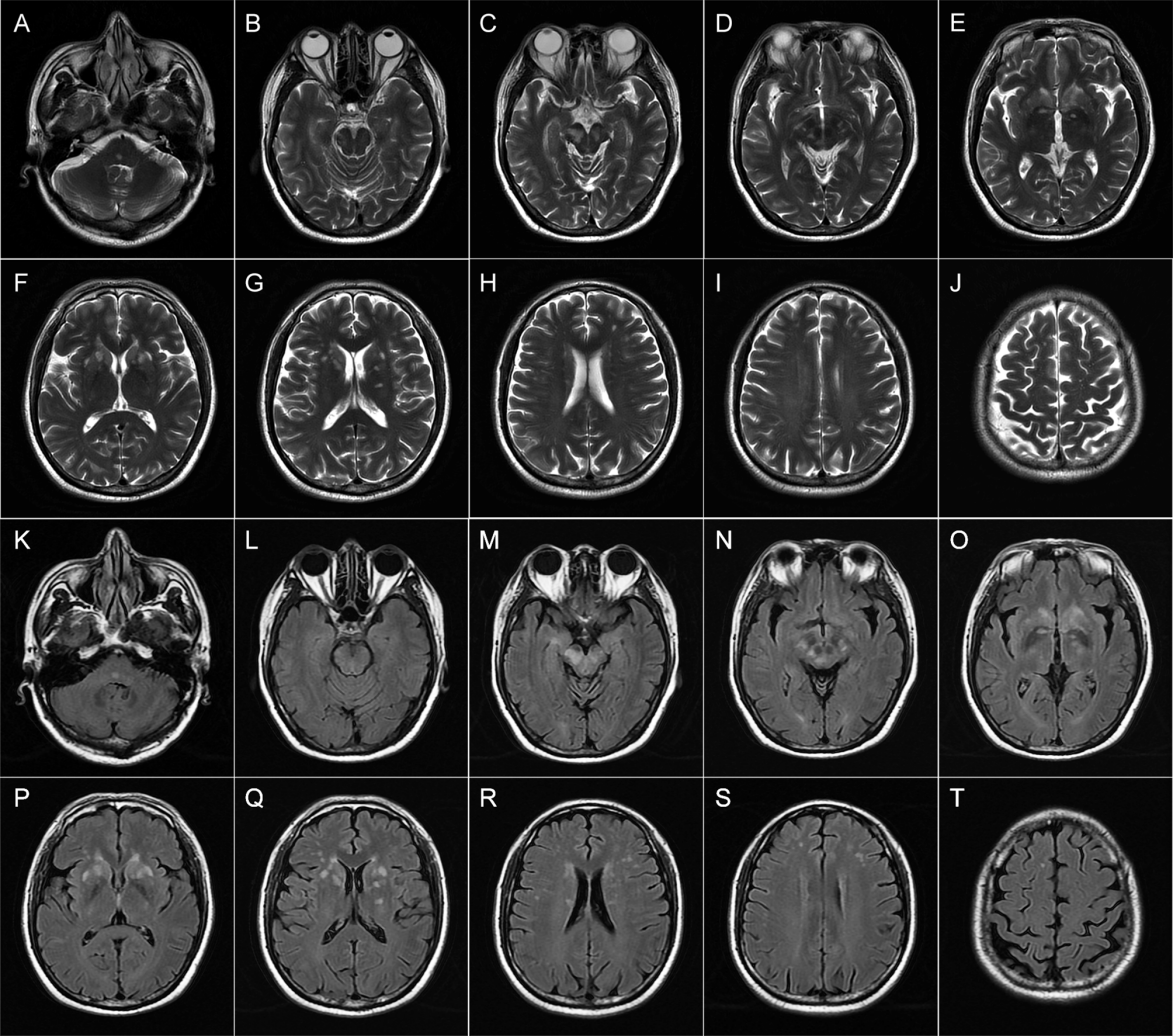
Brain magnetic resonance imaging (MRI) of patient 1 performed during acute attack. Axial T2-weighted (A–J) and FLAIR (K–T) images show multiple patchy hyperintense lesions in the tegmentum of the pons (A, K), bilateral midbrain (B–D, L–N), and right hippocampus (C, M); ovoid lesions with well-defined borders in the bilateral head of the caudate nucleus and putamen (E–G, O–Q); and spotty lesions involving bilateral paraventricular white matters (H, R) and subcortical white matters of the frontal lobes (H–J, R–T).
